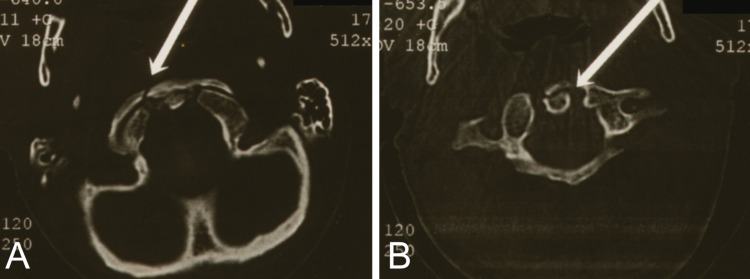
Computed tomography scans (A) Fracture of the anterior arch (white arrow) of the atlas and (B) coronal vertical fracture (white arrow) of the dens in a 76-year-old male.
